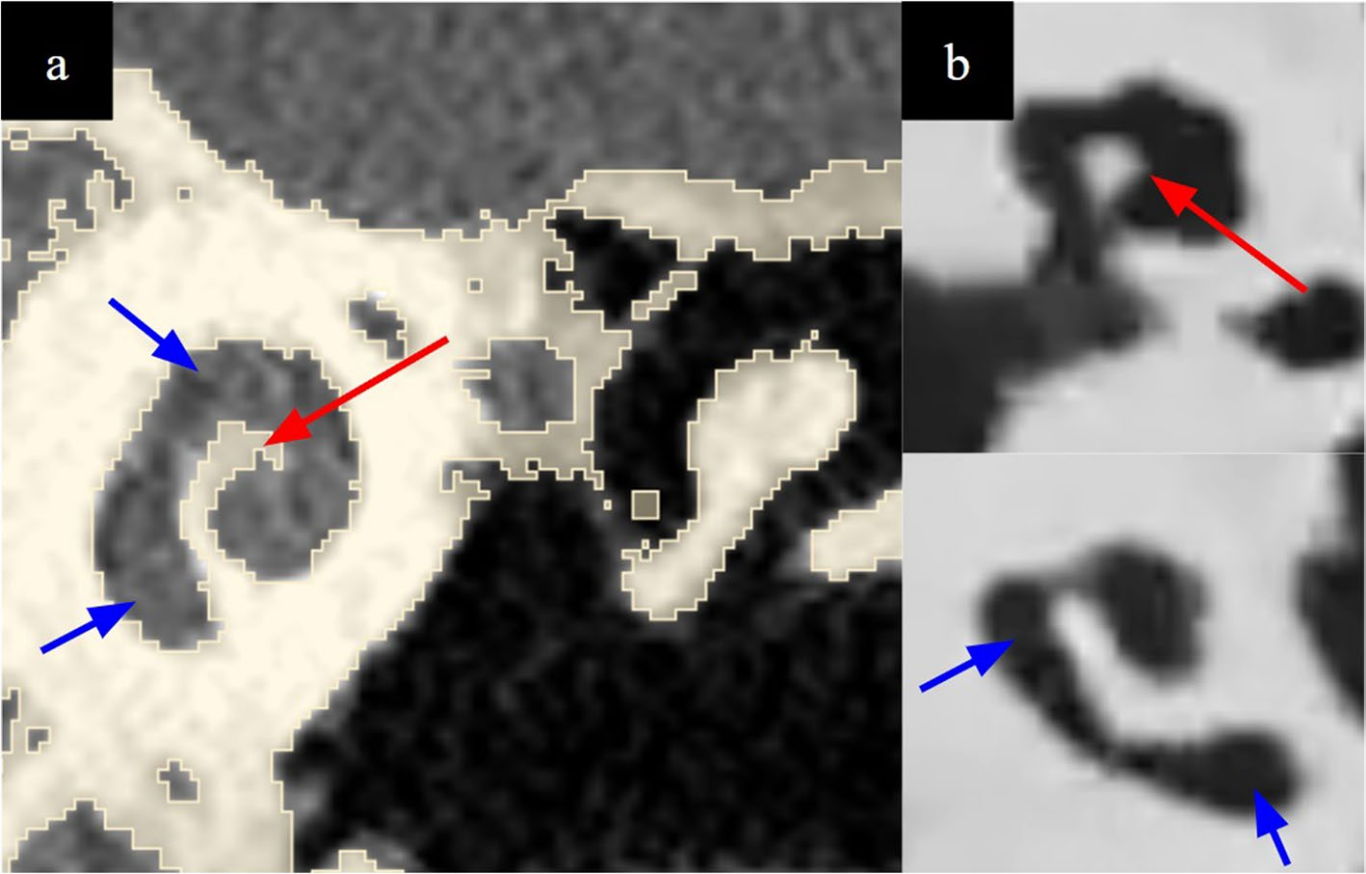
a The scala tympani (blue arrow) and modiolus (red arrow) on segmentation renderings and (b) concomitant CT imaging of the model
